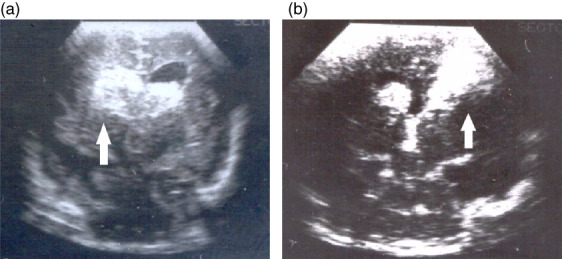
Grades of intraventricular haemorrhage. (a) Grade 3 intraventricular haemorrhage (IVH). Coronal view ultrasound scan showing the lateral ventricles (black) are greatly enlarged and full of clotted blood (white and arrowed). (b) Grade 4 IVH. Coronal view. In addition to intraventricular blood there is a white area of haemorrhagic infarction (white and arrowed).
